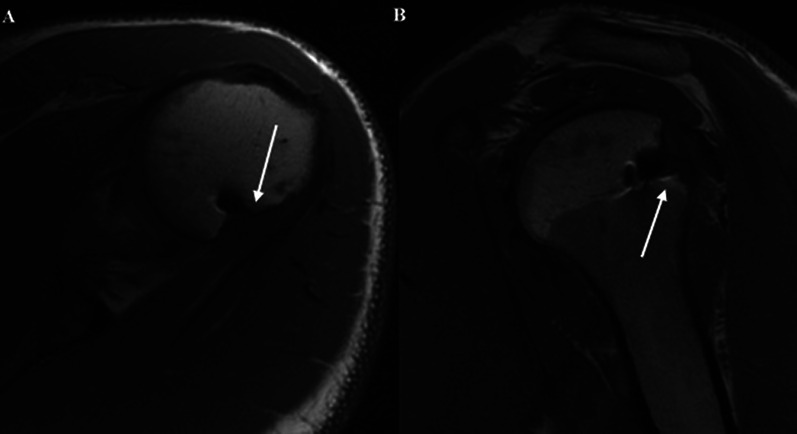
MRI axial A and sagittal B cuts of the shoulder of a patient who underwent the remplissage procedure. The* white arrows* show complete filling of the Hill–Sachs lesion in both the axial A and the sagittal B cuts
